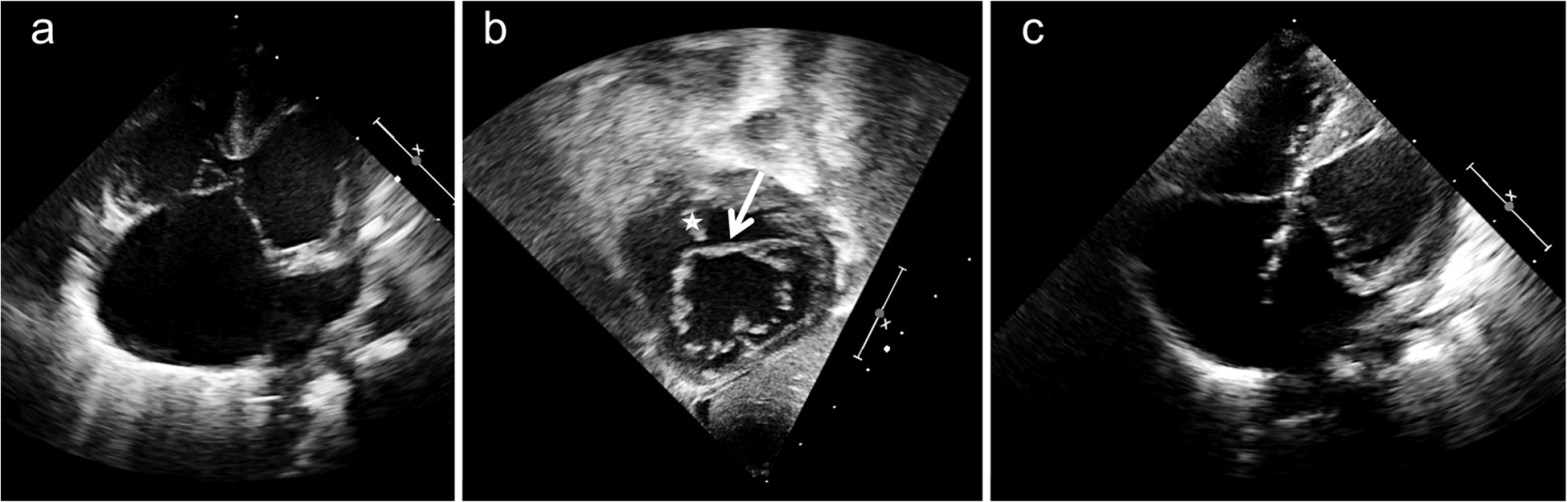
In patient 2 the apical 4-chamber view demonstrates UBC with absent left atrioventricular connection and balanced ventricles (a) (Video 1). The subcostal LAO view reveals a common atrioventricular valve with superior (arrow) and inferior bridging leaflets (b) (Video 2). The ventricular septum is marked by an asterix. Following surgery the apical 4-chamber shows successful septation of the common atrioventricular valve (c)
